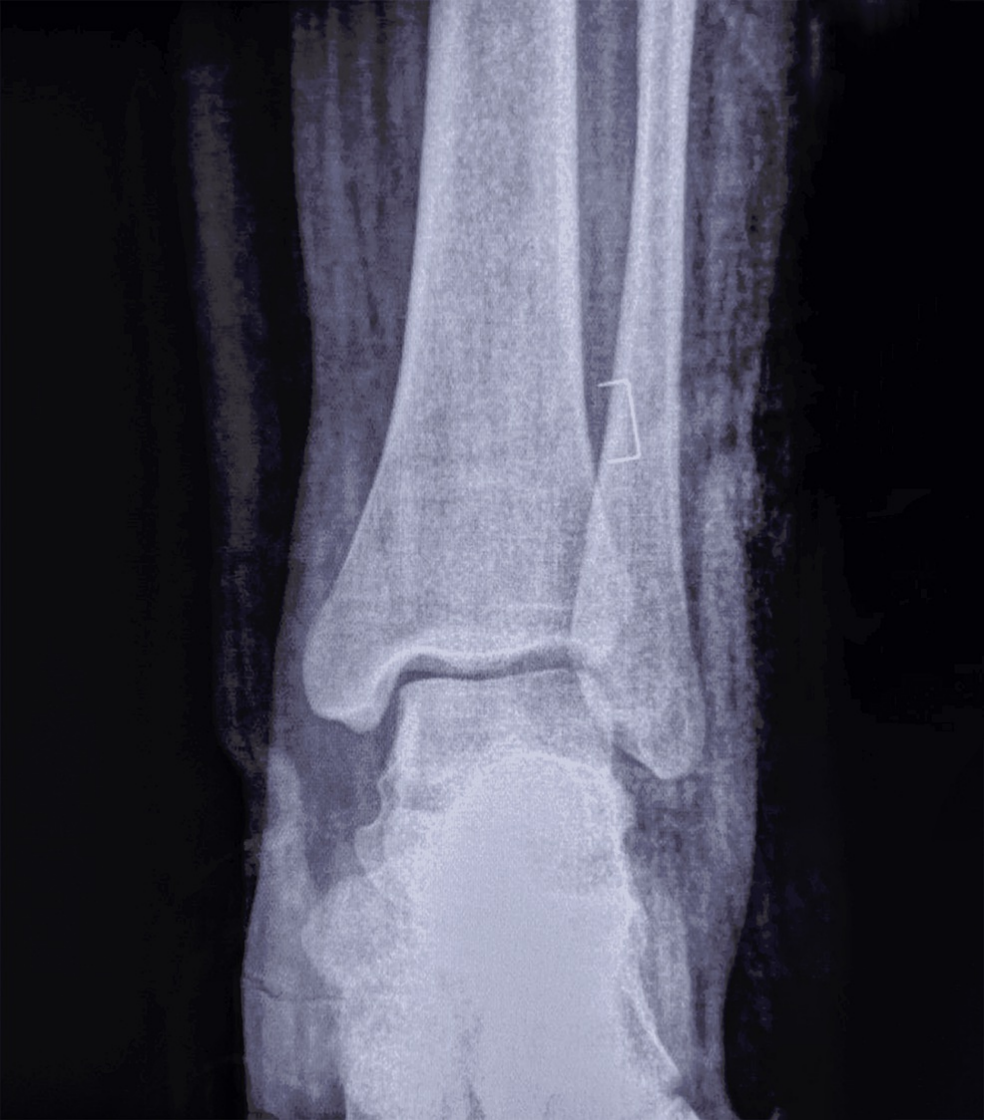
Anteroposterior post-reduction radiographic view of the left ankle.
